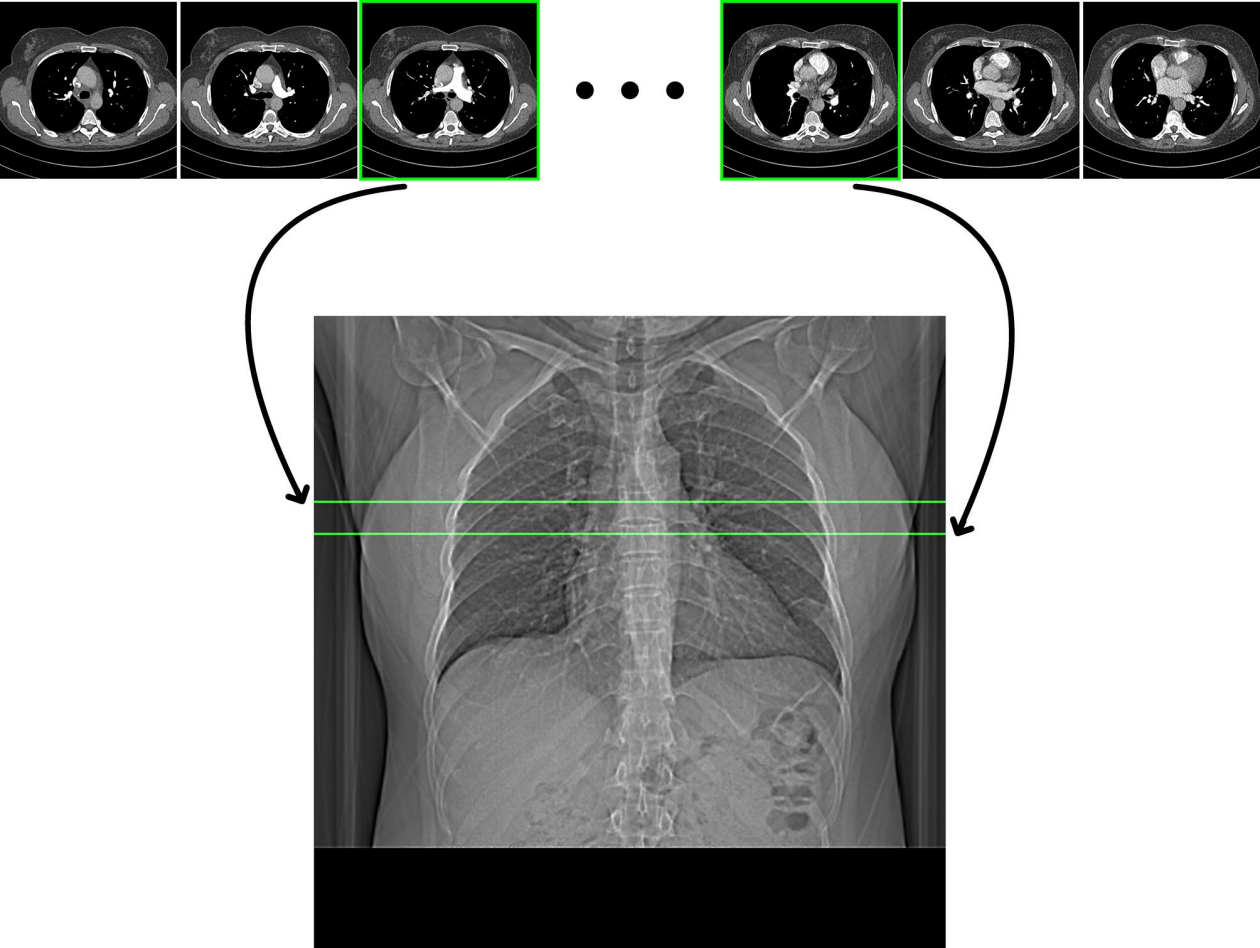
The axial slices of the CT scan between the cranial and caudal boundary of the pulmonary trunk were used to single out the 2D region in the CT scout view corresponding to the area of the pulmonary trunk (green). Images were cropped for better visibility.
